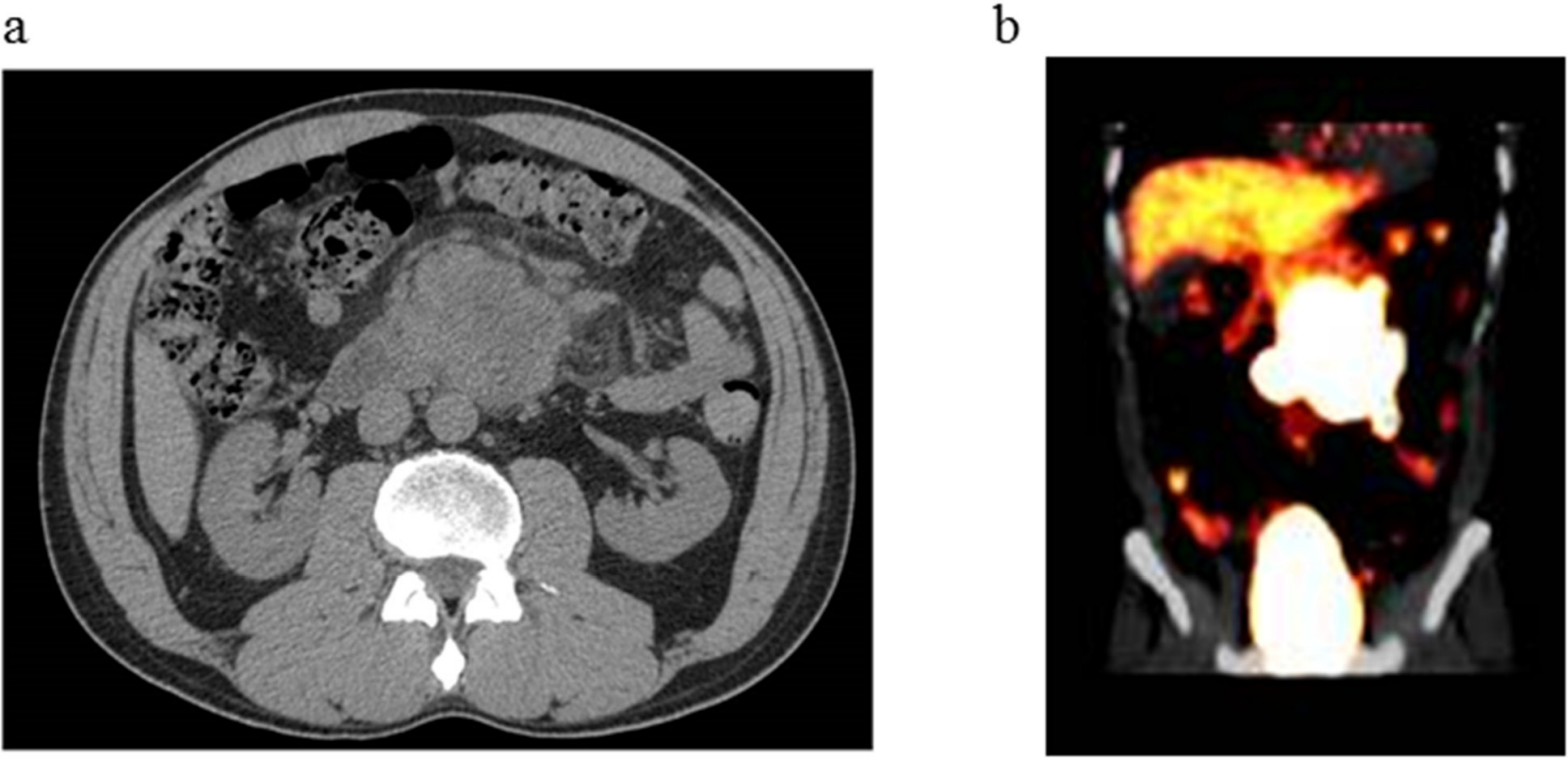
Radiological images of abdominal tumor. (a) Computed tomography revealed a 6 cm tumor at the duodenum. (b) Positron emission tomography showed a significant uptake at the tumor.
